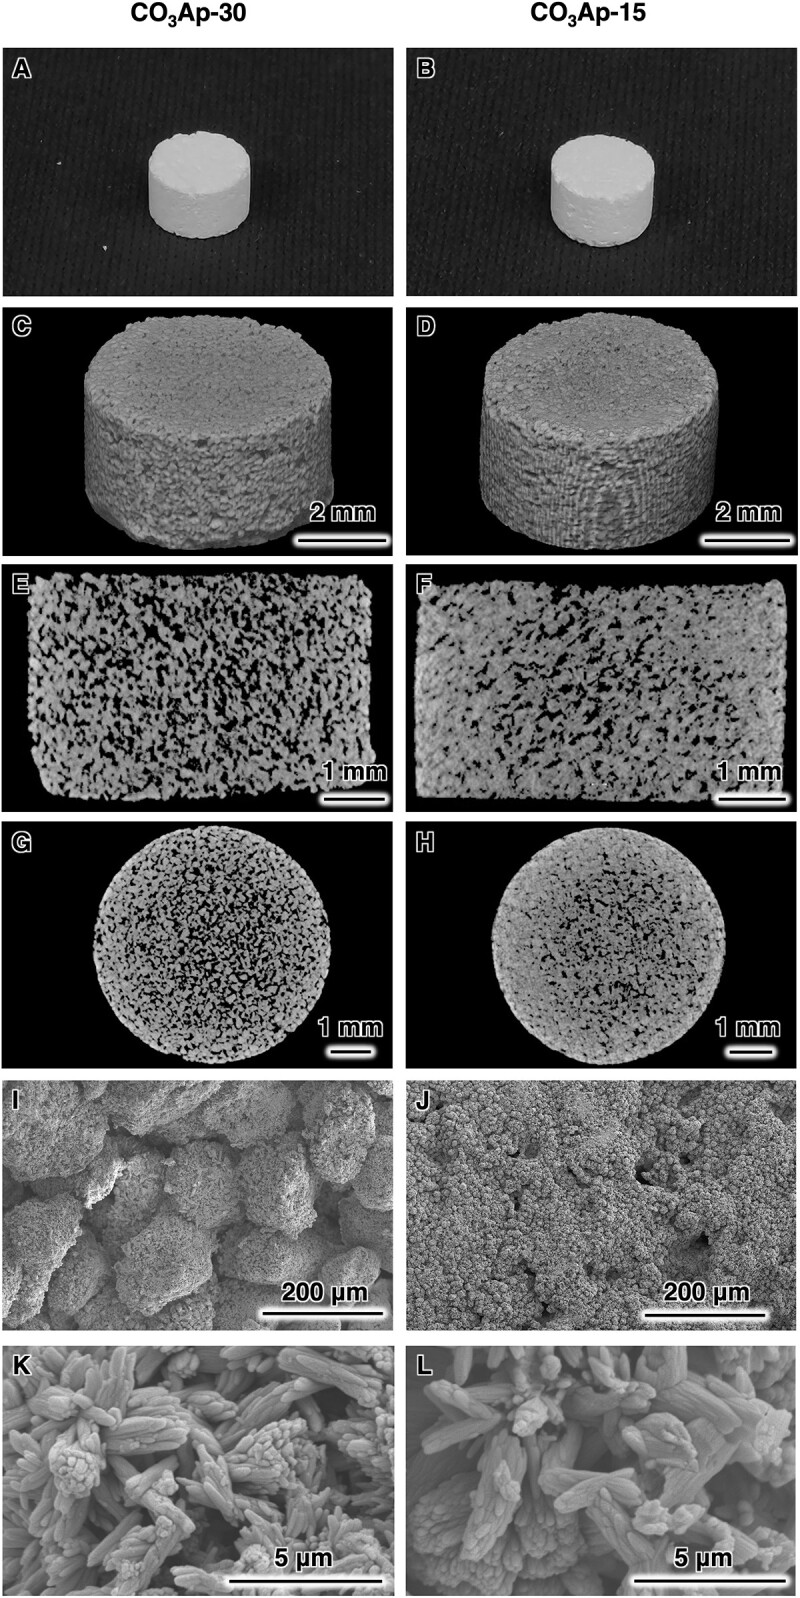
Photograph images of (A) CO_3_Ap-30 and (B) CO_3_Ap-15. μ-CT scanning observations of materials. μ-CT-based 3D reconstruction images of (C) CO_3_Ap-30 and (D) CO_3_Ap-15. μ-CT-based sliced images at a sagittal section of (E) CO_3_Ap-30 and (F) CO_3_Ap-15. μ-CT-based sliced images at a transverse section of (G) CO_3_Ap-30 and (H) CO_3_Ap-15. SEM images of (I) CO_3_Ap-30 and (J) CO_3_Ap-15. Magnified SEM images of (K) CO_3_Ap-30 and (L) CO_3_Ap-15.
